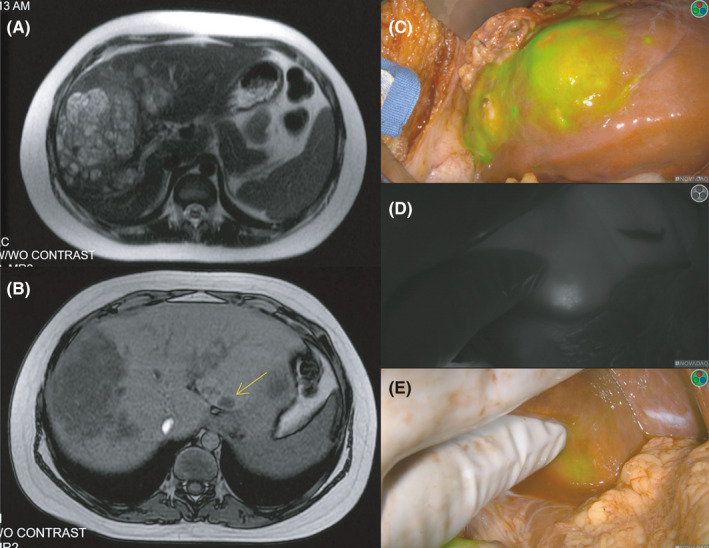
CT and intraoperative imaging of ICG‐positive abdominal lesions. The left panel (A‐B) demonstrates the preoperative CT in axial views. Panel a shows the T2‐weighted image of the right sided lesions; Panel b shows the multifocality of this tumor indicated by the yellow arrow in the T1‐weighted image. The right (C‐E) panel shows the intraoperative imaging during laparotomy using the Pinpoint ICG system. Panel c demonstrates the ICG overlay of the large right‐sided lesion. Panel d and e exhibit the ICG‐avidity of a smaller left‐sided lesion with the black and white (D) and ICG overlay (E)
